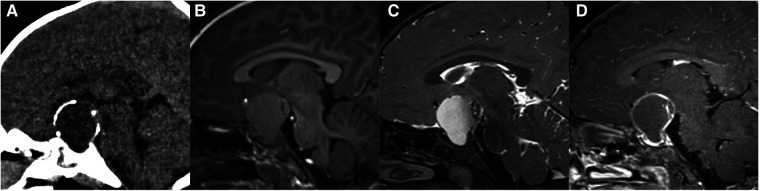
29-month-old girl with craniopharyngioma: sagittal unenhanced CT (A), T1WI (B), T2WI (C), and post-contrast T1WI (D) show a cystic mass with eggshell calcification in the sellar and suprasellar region.
